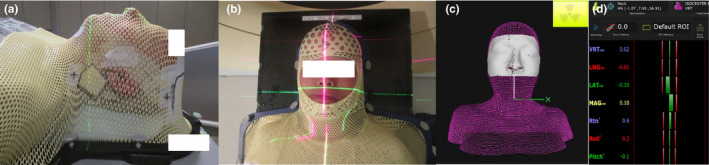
(a) Non‐surface guided radiation therapy (SGRT) setup: A conventional closed thermoplastic S‐frame mask molded on the patient to cover the head and shoulder regions. Marks are placed on the mask to align with the lasers for patient setup, (b) SGRT setup: An open S‐frame mask was used with the mid‐face opening and the ROI was set in the area for SGRT monitoring, (c) SGRT monitoring: the surface rendering, generated from the CT dataset in the SGRT system to assist with daily patient setup, (d) SGRT tolerance: SGRT patients were first set up with optical image guidance to ensure that the isocenter was within the 2 mm/2° tolerance. On‐board imaging (kV‐kV/cone‐beam CT) was subsequently performed to verify and correct patient alignment.
